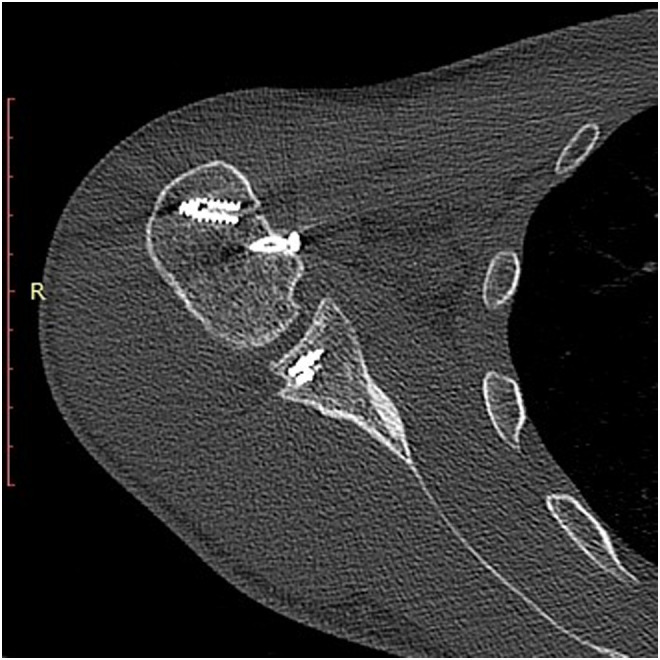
1st patient: Postoperative CT scan of Right shoulder, after the second operation of arthroscopy and reverse Bankart repair. After addressing both sides of the joint, the humeral head is centralized in the glenoid.
